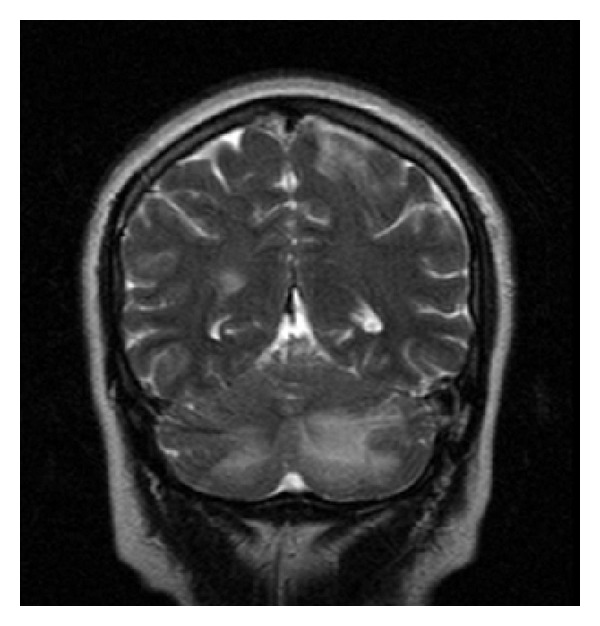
MRI, T_2_-weighted coronal image: hyperintense signal on bilateral occipital and cerebellar regions.
